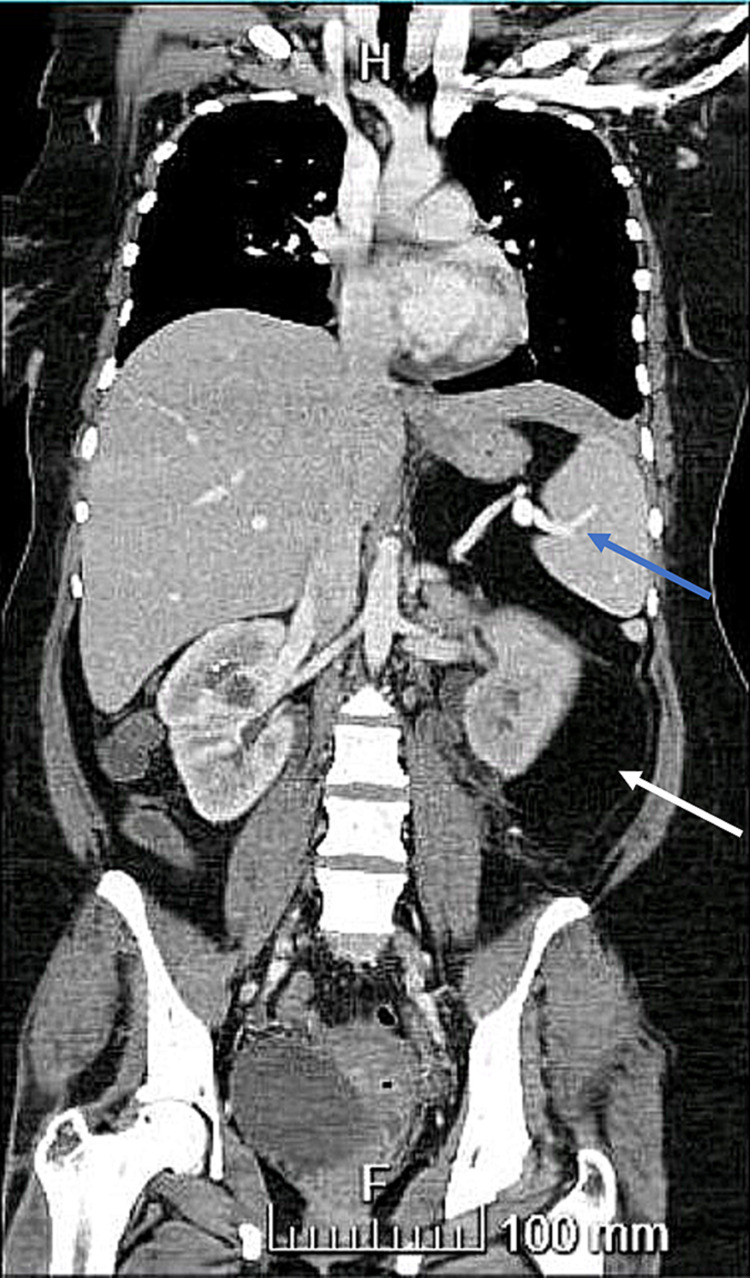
CT chest, abdomen, and pelvis for staging: large fat-containing lesion (white arrow) seen intra-abdominally extending from the left upper quadrant. The splenic artery and vein run within the lesion centrally to the splenic hilum (blue arrow) with no compression, infiltration, thrombosis, or occlusion.
